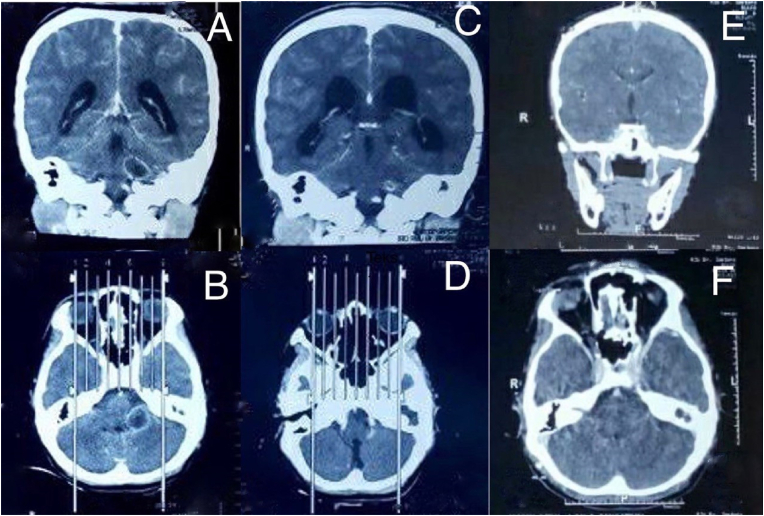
Case 1. A, B Initial CT head scan with contrast. C, D CT head scan at the third week of antibiotic administration. E, F CT head scan at the sixth week of antibiotic administration.
